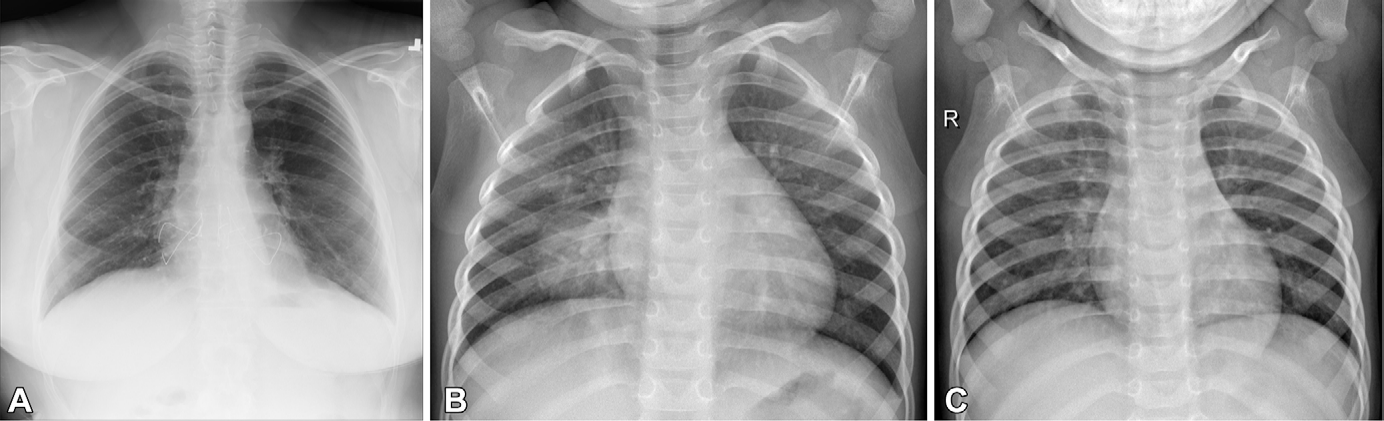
Three example images from the chest radiograph dataset. (A)
